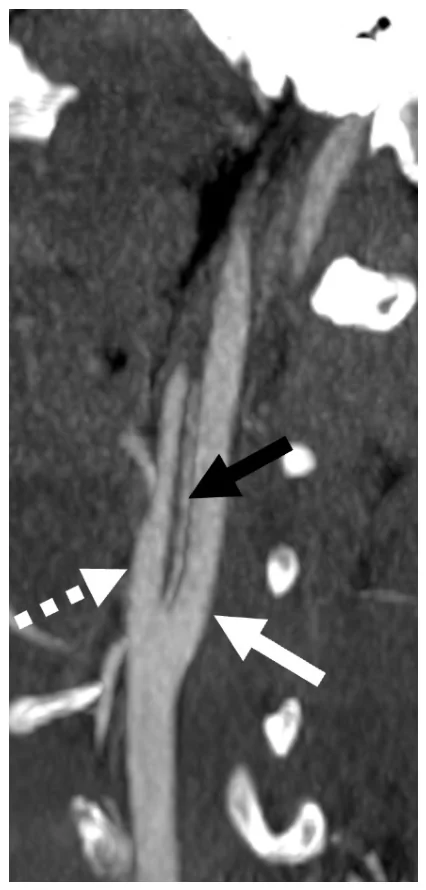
Carotid trifurcation. Sagittal MIP CTA shows carotid trifurcation into the ECA (dashed white arrow), ICA (white arrow), and ascending pharyngeal artery (black arrow).
